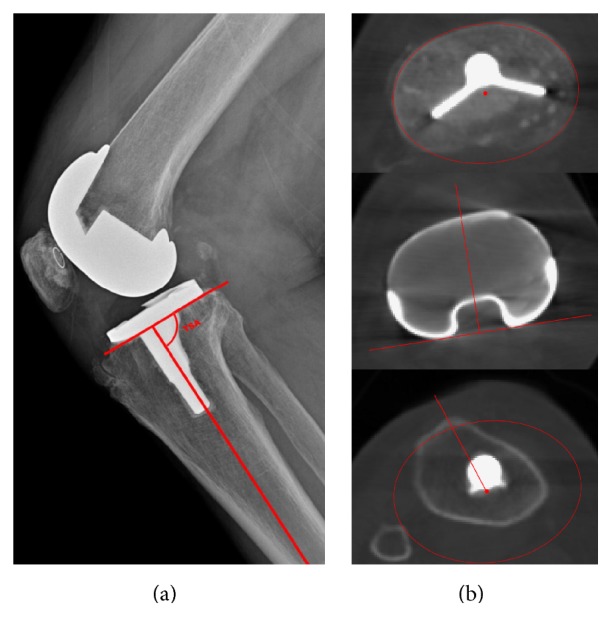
The method for measurement of (a) the tibial sagittal angle and (b) the tibial component rotation angle from CT images.
